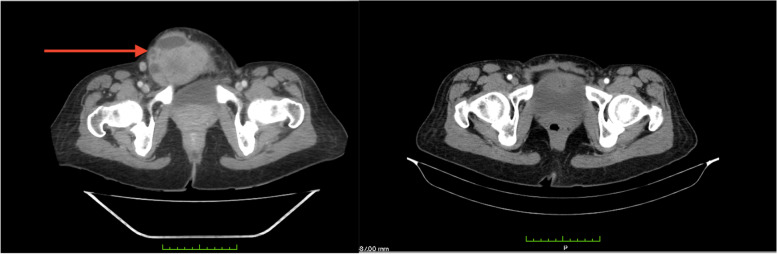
Axial CT images of the abdomen and pelvis. A Before resection. B After surgery (resection of the abdominal wall mass, hysterectomy, bilateral salpingo-oophorectomy, and closure with polypropylene mesh
